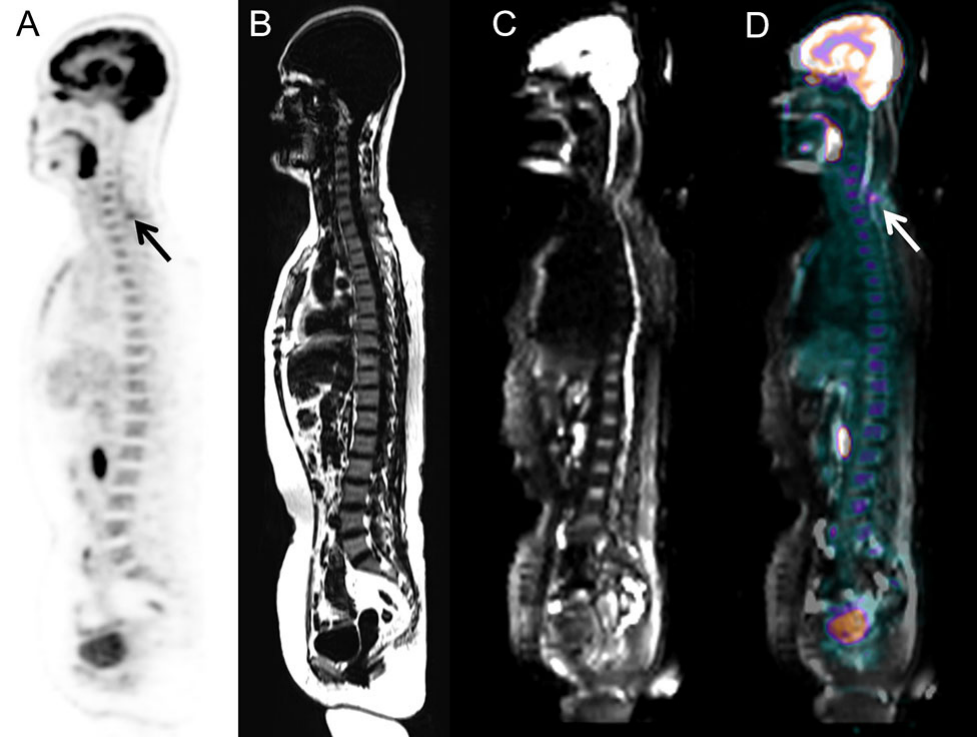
Images of a 58-year-old woman with low-grade follicular lymphoma. BMI was detected via PET/MRI according to the unifocal intense uptake of FDG on the PET image, while MRI did not show any intense signal because of artifacts. The presence of BMI was also confirmed through BMB. (A) PET image derived from integrated PET/MRI. (B) TIWI image derived from integrated PET/MRI. (C) DWI image derived from integrated PET/MRI. (D) Fused whole-body integrated PET/MRI.
